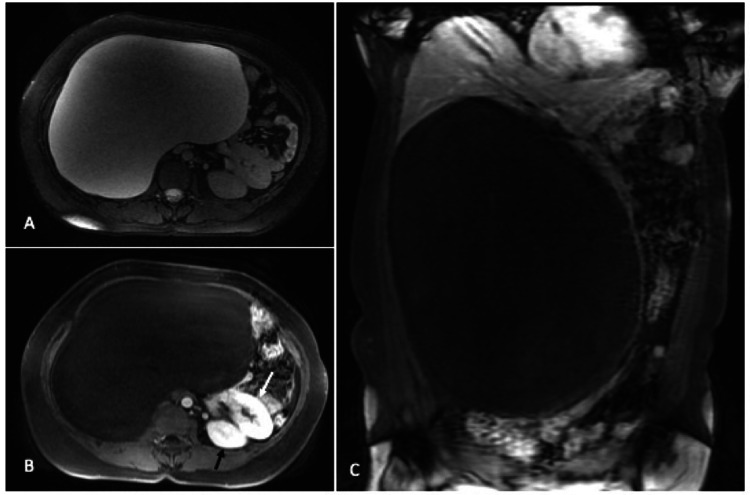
T2W axial (A) and fat-saturated T1W post-contrast axial (B) and coronal (C) MRI images reveal the giant right adrenal cyst with no evidence of septations or any enhancing solid component The displaced right kidney (white arrow in B) is seen anteroinferior to the left kidney (black arrow).
